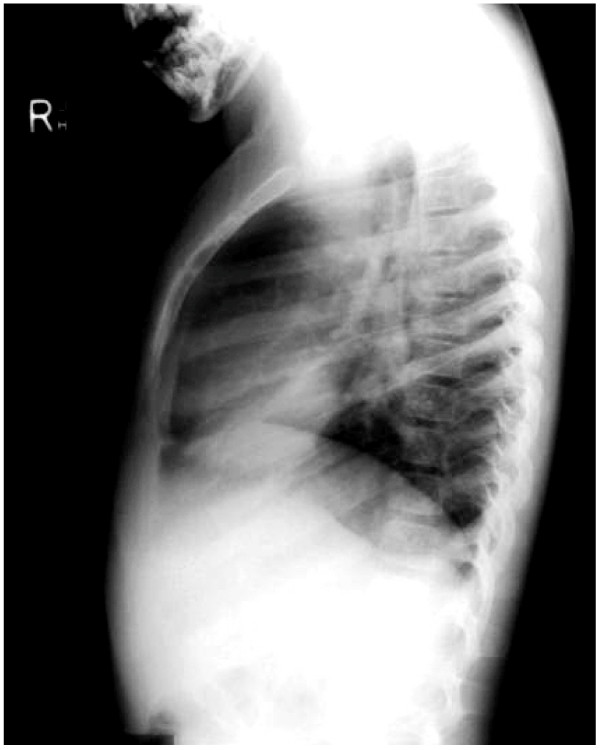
Lateral plain radiograph Turner syndrome scoliosis with hypokyphosis.
